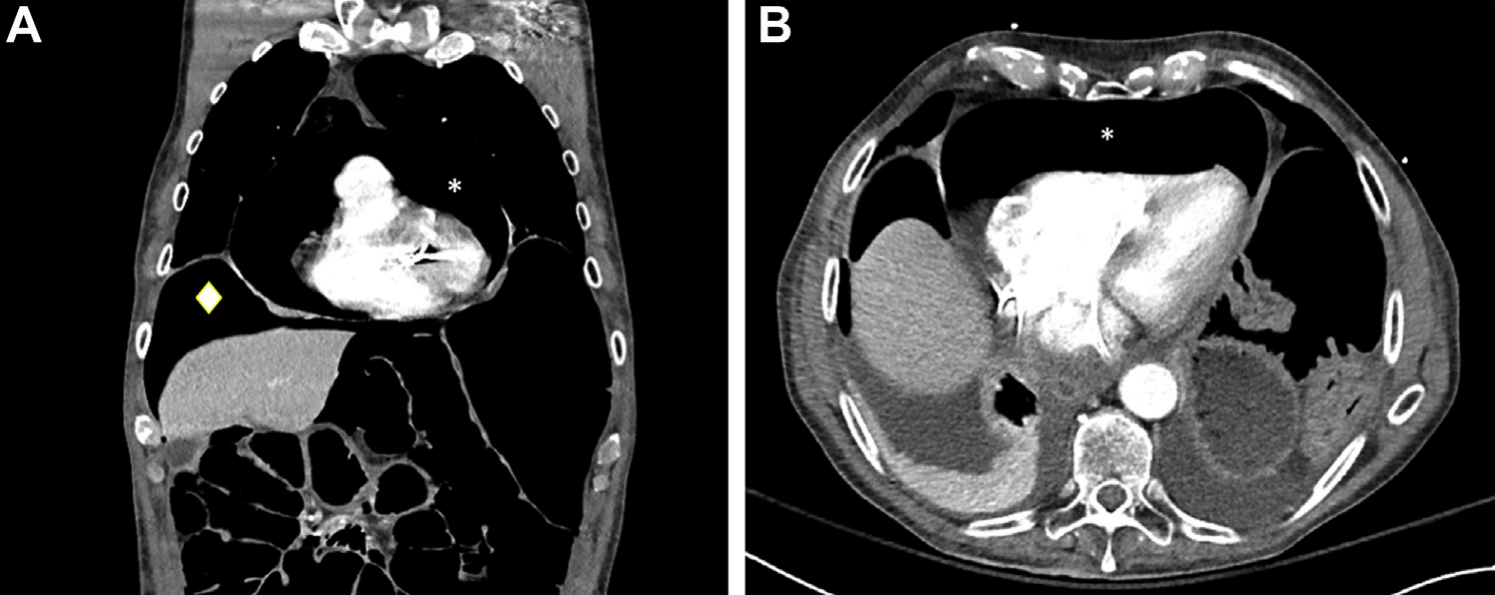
Further CT Imaging (A) Computed tomography (CT) scan showing a large pneumopericardium (asterisk) and pneumoperitoneum (diamond). (B) Computed tomography scan showing large pneumopericardium (asterisk).
